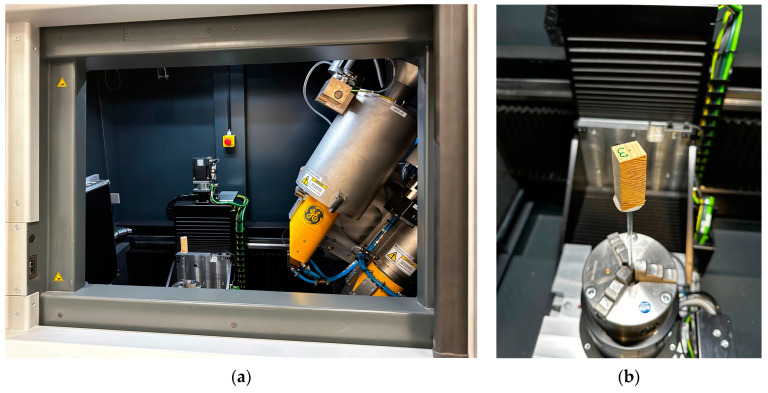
X-ray computed tomography studies: (a) GE Phoenix v-tome-x m device during the examination; (b) a sample prepared for tomographic studies mounted inside the device.
